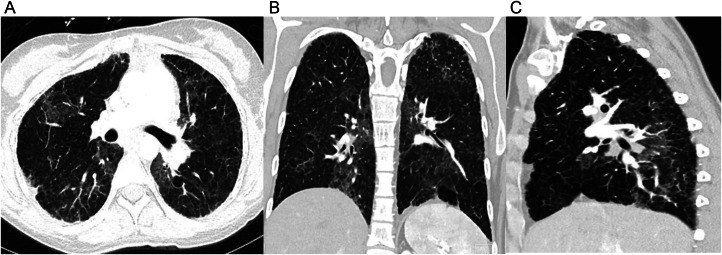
Imaging findings at 26 years of age (case 2). Axial (A), coronal (B), and sagittal (C) chest CT images show patchy ground glass opacities with interlobular septal thickening (crazy paving), small thin-walled cysts, and mild peripheral reticulations.
